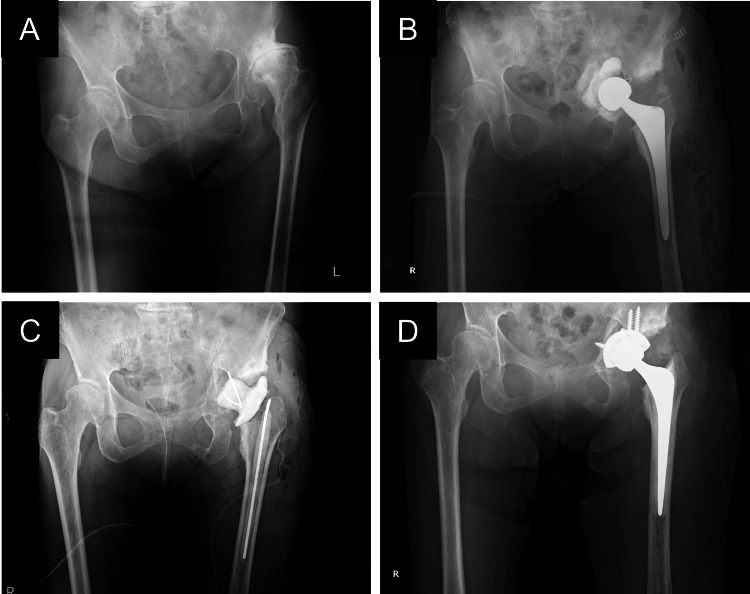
X-ray images of the hip A, before primary THA; B, before first-stage removal; C, after first-stage removal; D, after second-stage reimplantation
